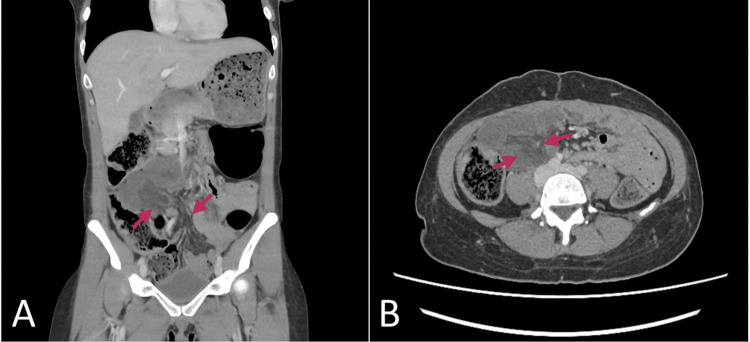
CT abdomen and pelvis with IV contrast, with (A) coronal and (B) axial views demonstrating minimal bowel wall edema and lack of contrast enhancement in the duodenum's region (arrows)
